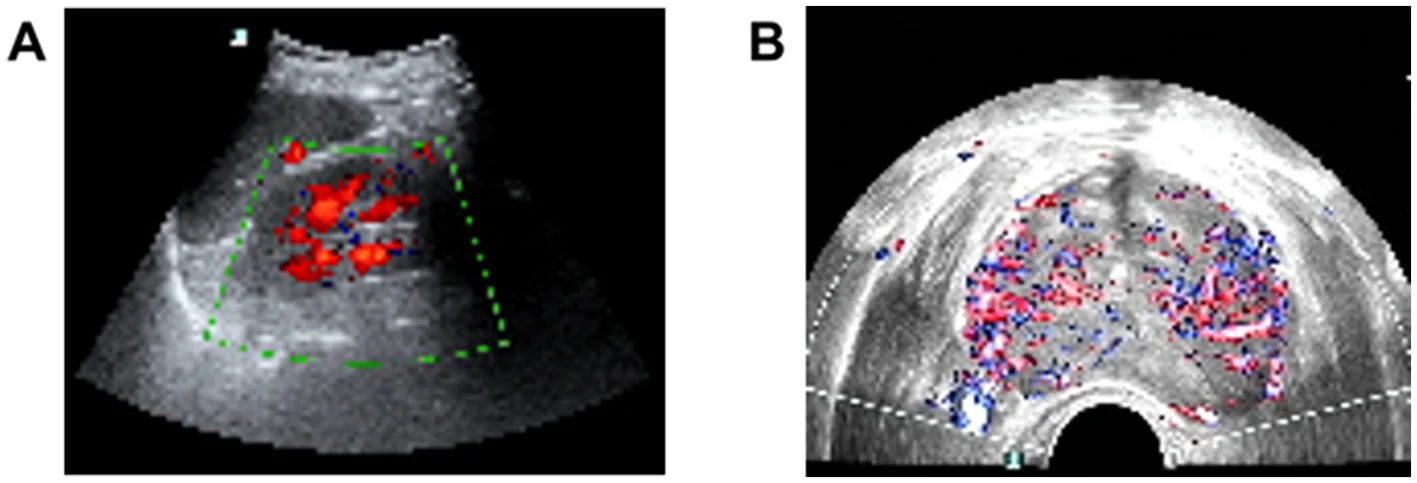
(A) Ultrasonography showed an enlarged prostate gland, and inhomogeneous echogenicity, (B) Transrectal elasticity and ultrasound of prostate.
